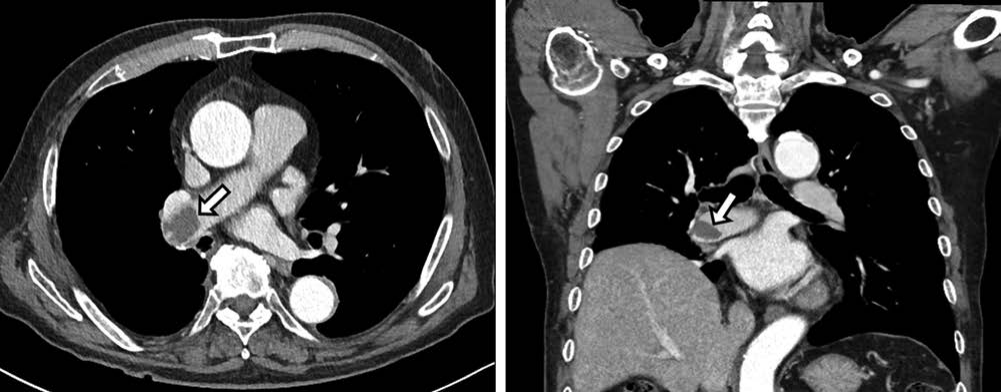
Chest computed tomographic images of pulmonary artery stump thrombosis. A filling defect (arrow) is observed in the right pulmonary artery after a right lower lobectomy.
